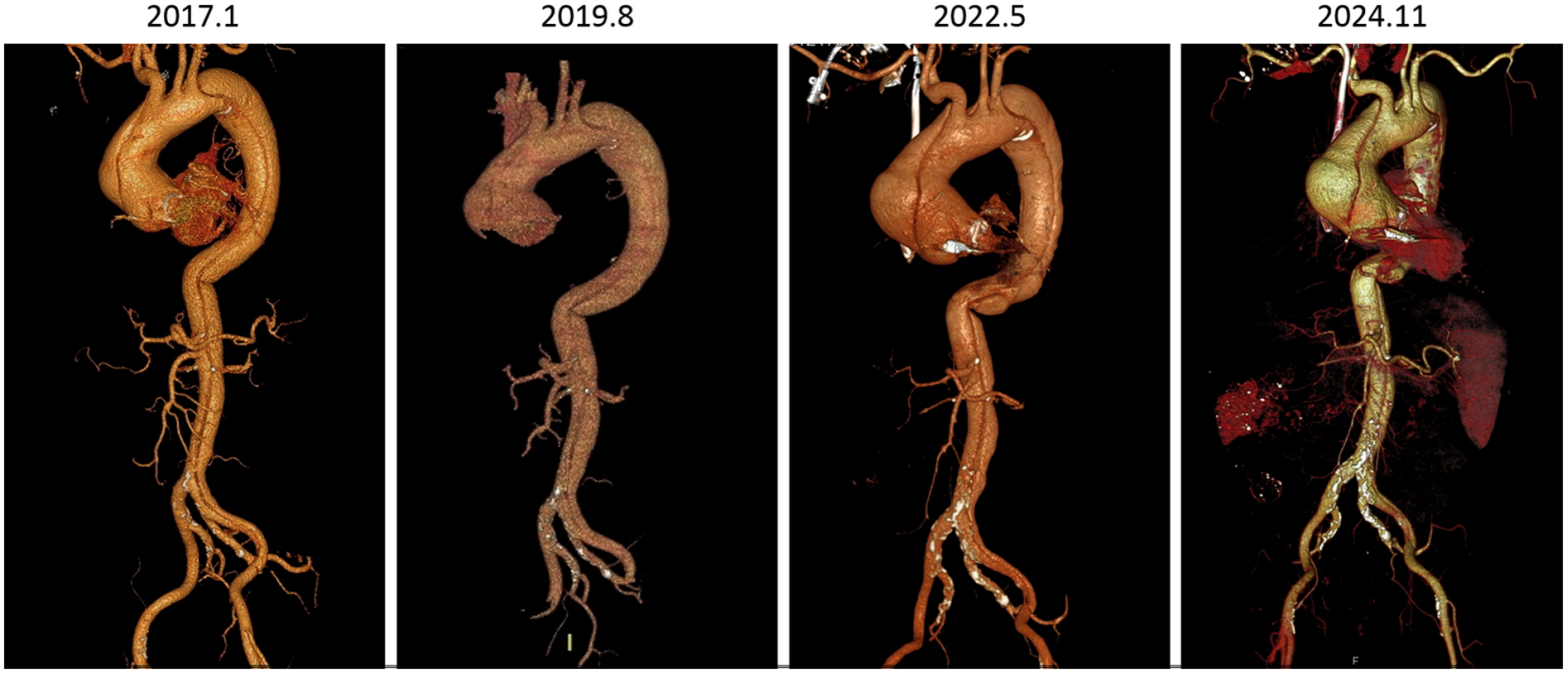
Computed tomography angiography images of different time points.
